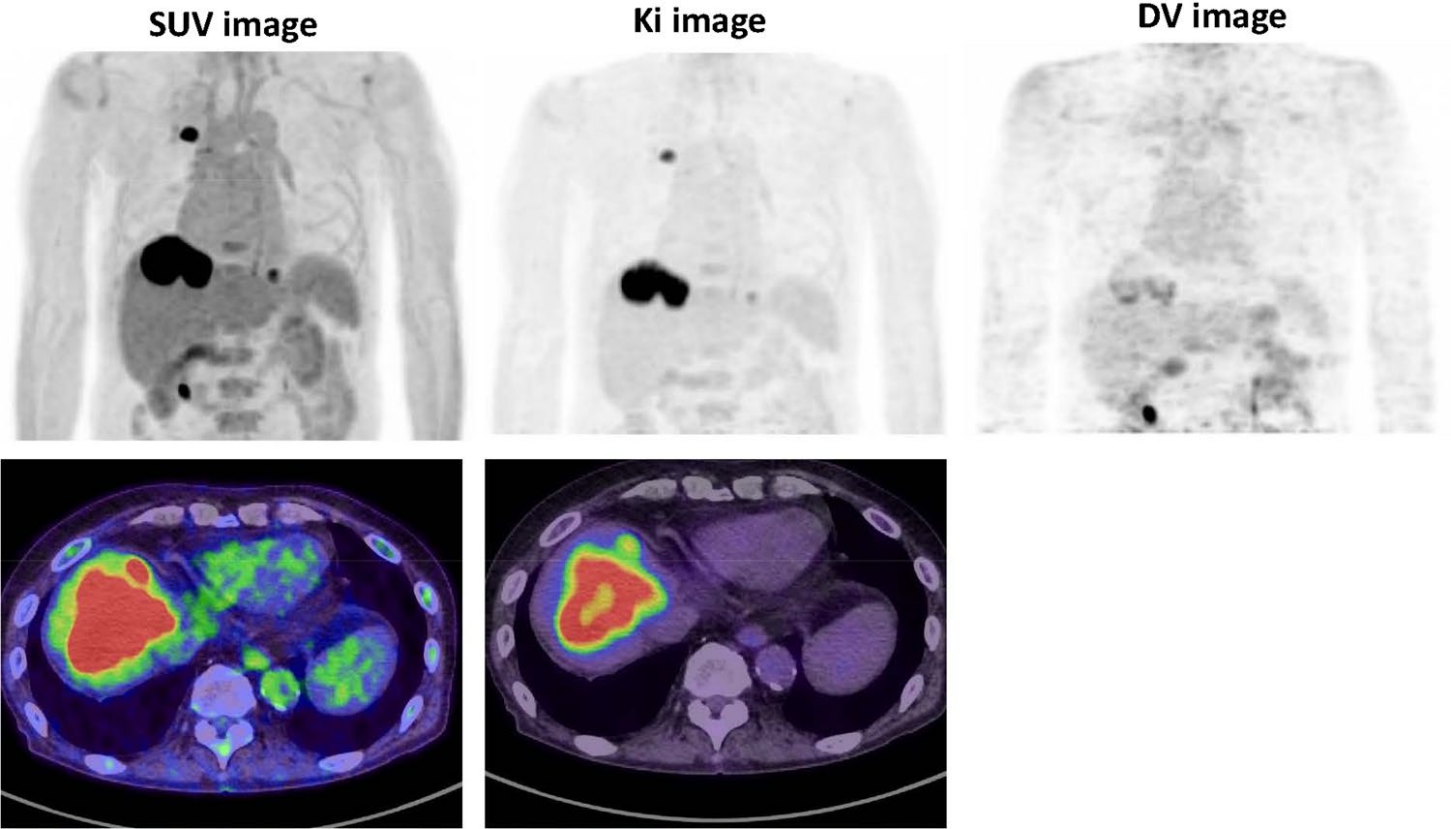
Whole-body (top) images of SUV, Ki, and distribution volume (DV) parameters estimated from the serial dynamic imaging as shown in Fig. 1. Axial images of the SUV and Ki are shown at the bottom. Higher contrast of the lesions was noted with attenuated physiologic uptake in the mediastinum and liver on Ki images
